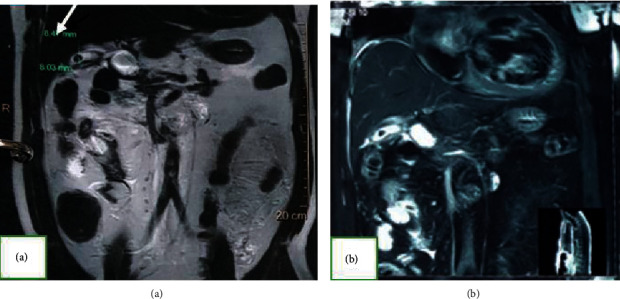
Abdominal MRI: (a) gall bladder with the stone inside (arrow) and free peritoneal fluid; (b) no dilatation of the intrahepatic bile duct (IHBD) and extra hepatic bile duct (EHBD).
